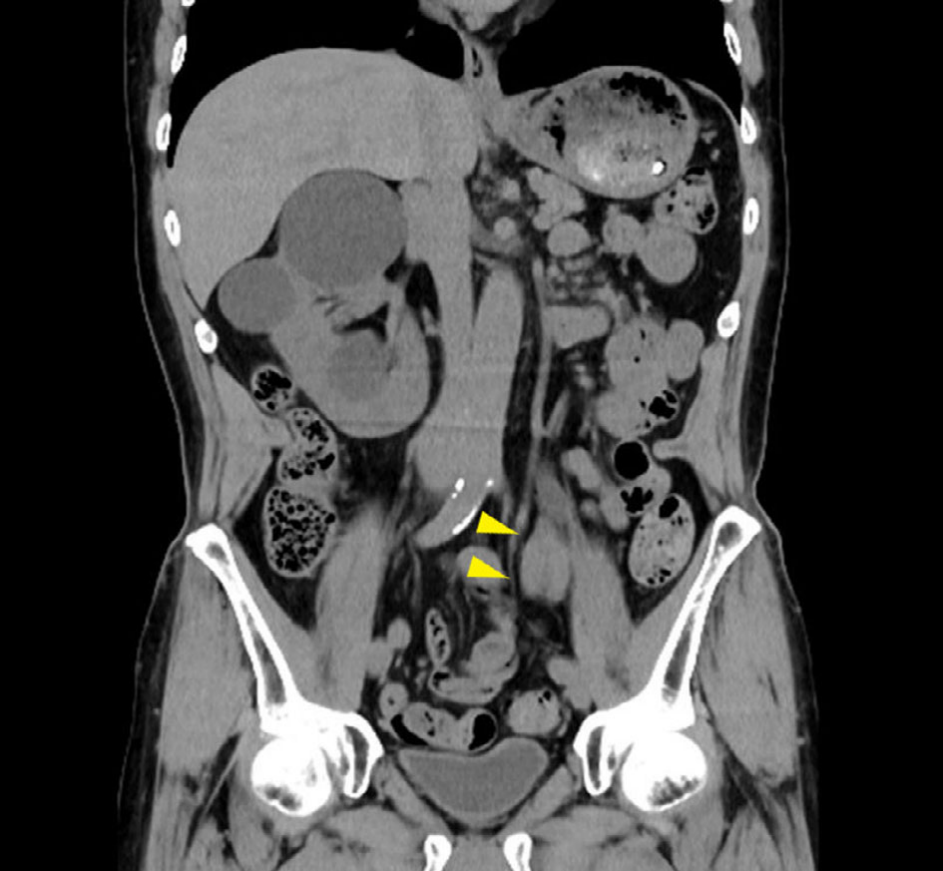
Computed tomography. A solid tumor measuring 41 × 23 mm was located on the left residual ureter (arrow).
